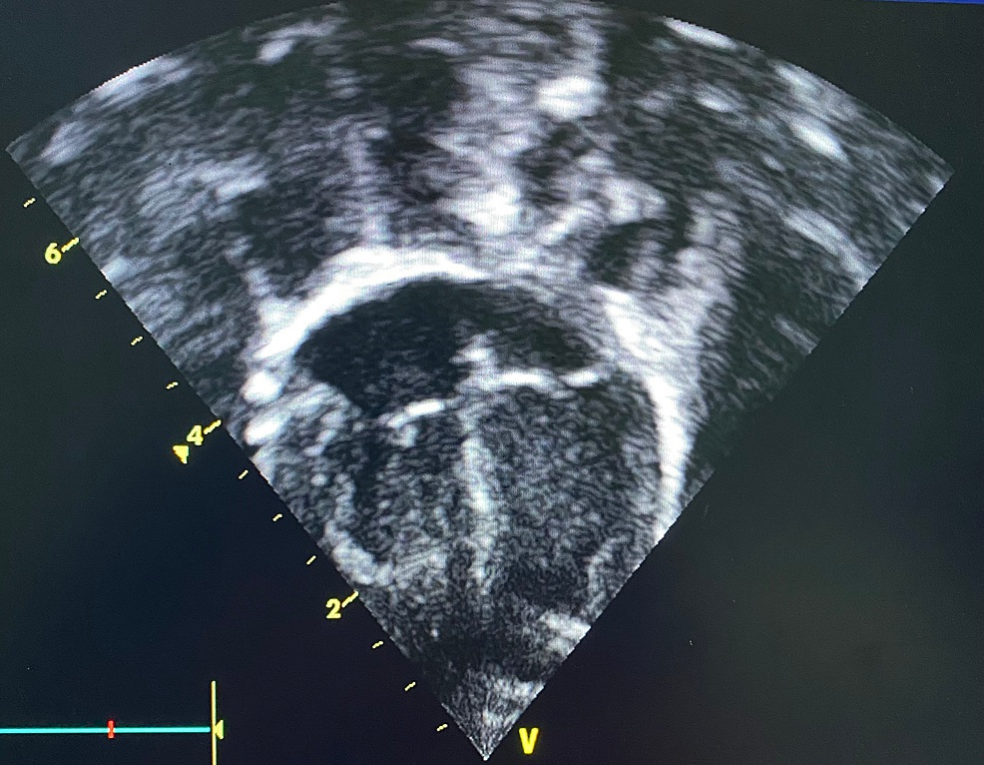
Echocardiogram, four-chamber view with normal findings without cardiac abnormality or fluid accumulation.
